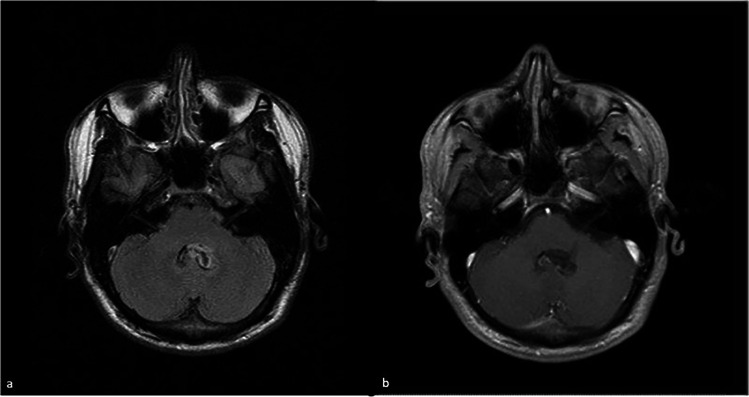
(a) Axial FLAIR MRI at the level of cerebellopontine pedincle reveals behavioral change of the lesion with appearance of a cystic component at the center and remanence of the ubiquitous hyperintensity on the edge of cyst. (b) Remaining gadolinium-enhancement at the solitary component of the lesion in the axial post-Gadolinium T1 weighted MRI
